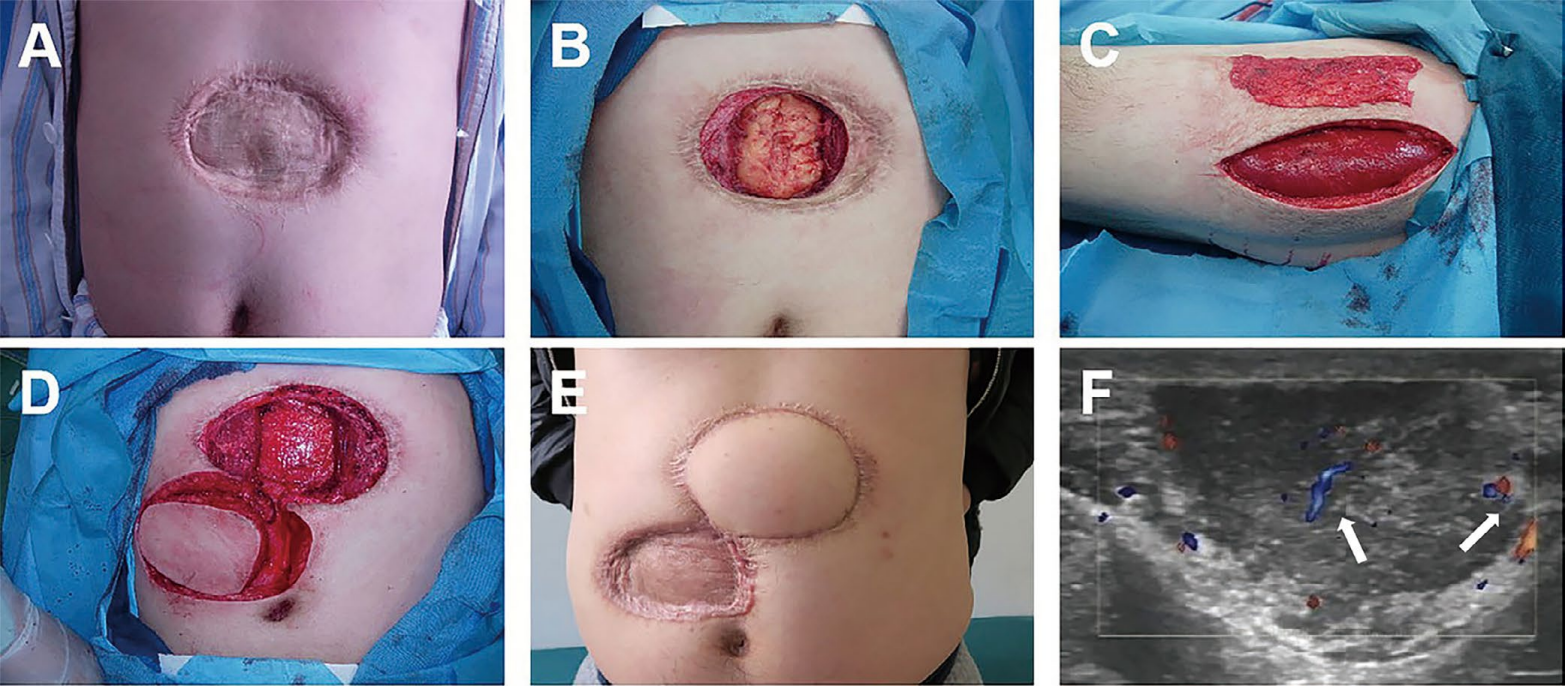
(A) A 37‐year‐old man with recurrent DFSP in the abdominal wall. (B) The peritoneum defect after removing the tumor. (C) Harvesting the broad fascia of the left thigh. (D) Harvesting the deep superior epigastric artery flap. (E) 6 months after the surgery. (F) Color Doppler ultrasonogram revealing rod‐shaped blood flow signals (white arrow).
